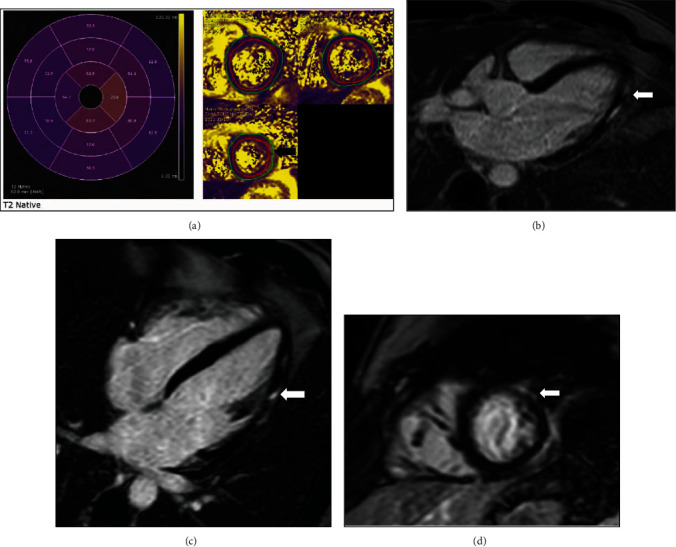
Patient D cardiac MRI: (a) T2 map demonstrating increased T2 signal consistent with edema in the epicardium of the apical lateral wall; (b–d) 3-chamber, 4-chamber, and apical short axis delayed images, respectively, demonstrating epicardial late gadolinium enhancement (LGE) corresponding to area of edema on T2 map.
